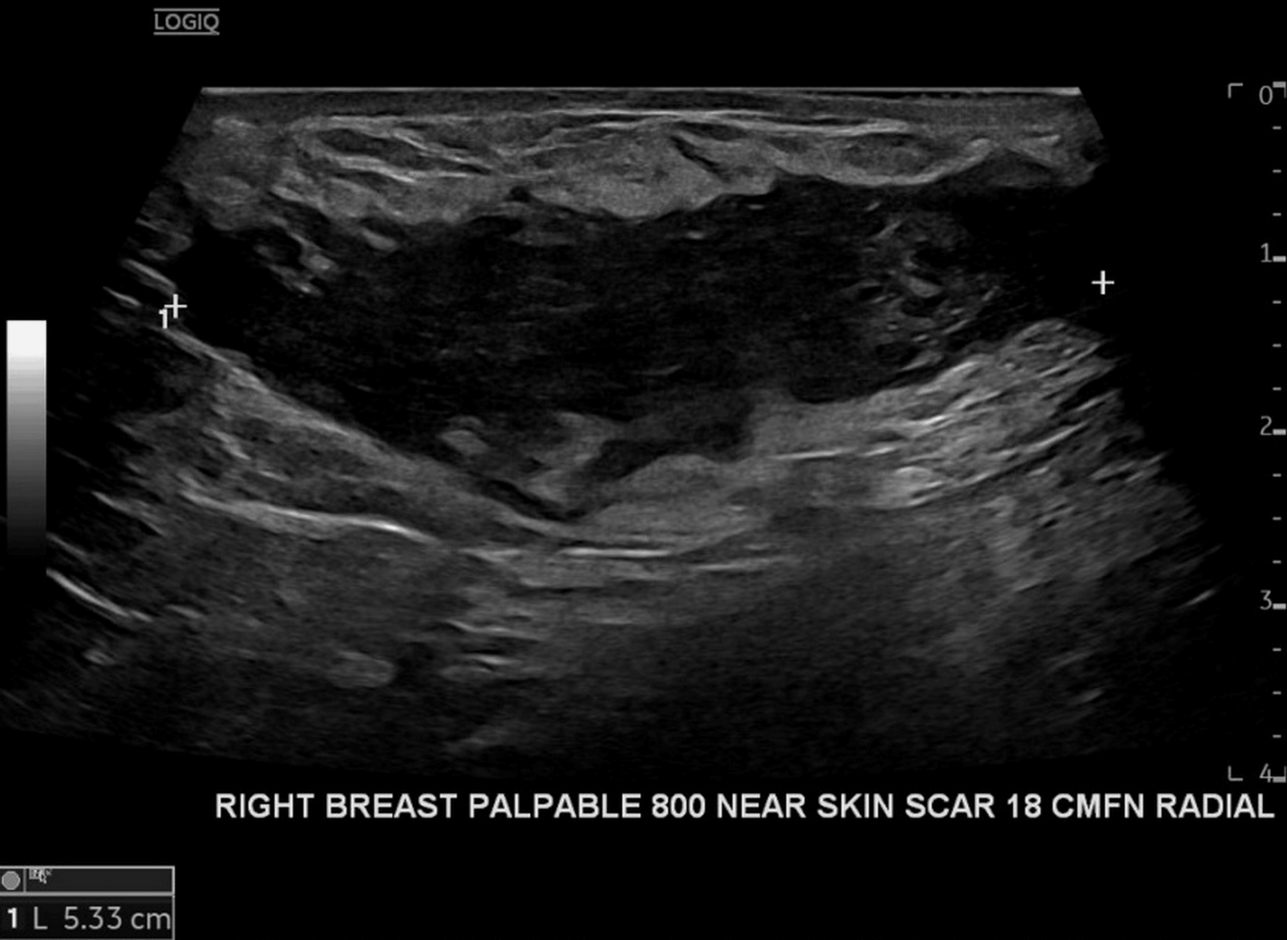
Right breast ultrasound This ultrasound view of the right breast mass at 8 o'clock demonstrates palpable breast mass
